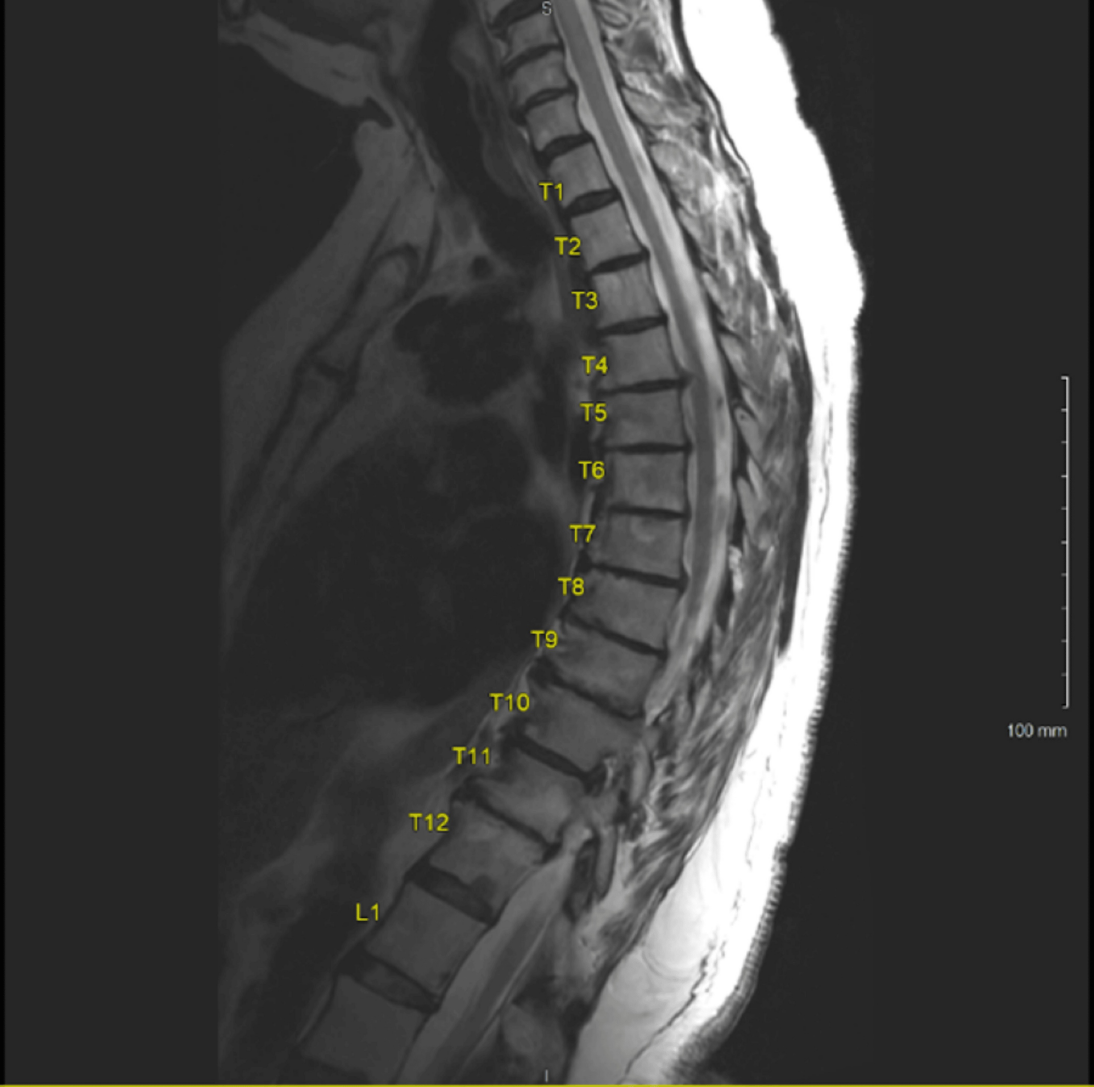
MRI of the thoracic spine with a spinal cord thoracic syrinx spanning T5 to T11
